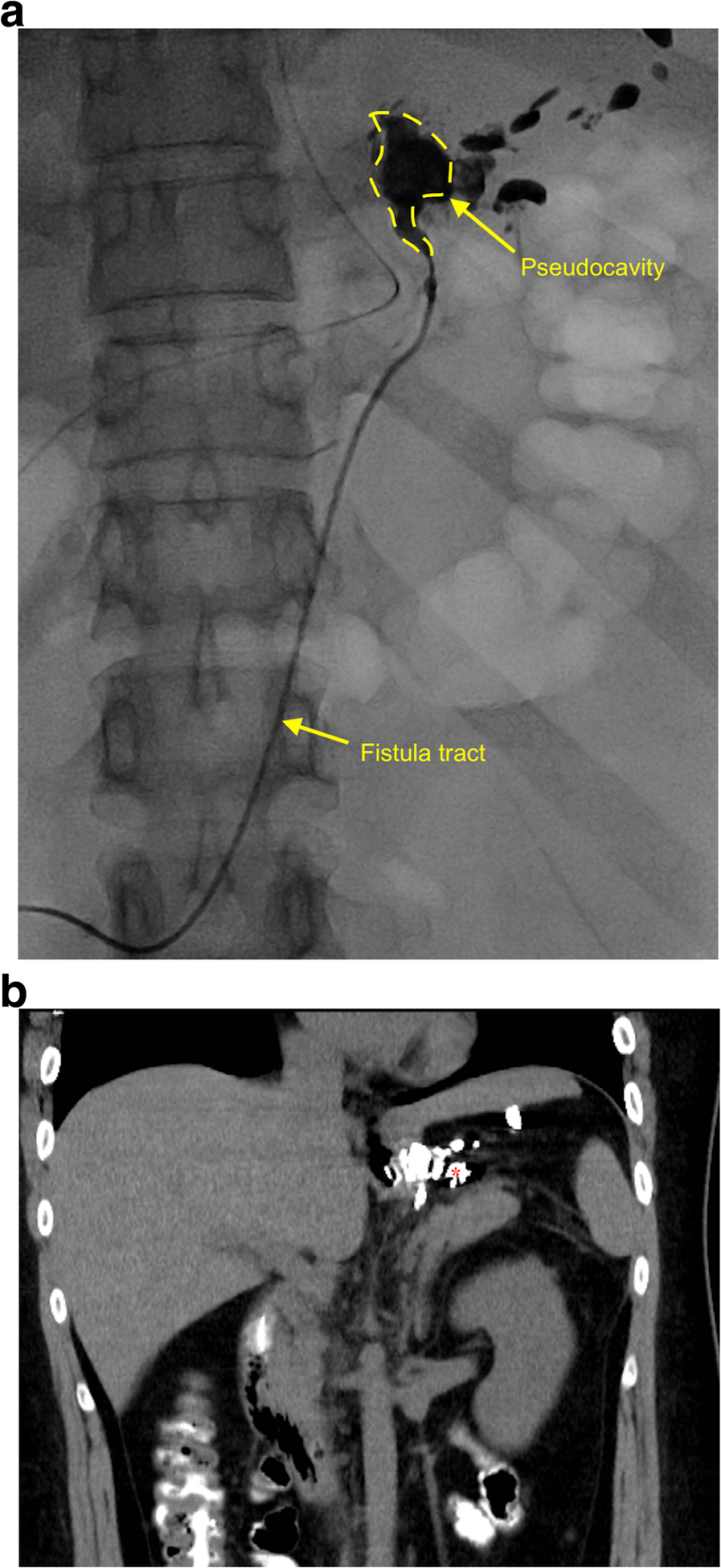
Percutaneous fistula tract embolization was performed through the CWV drain insertion site at POD63 (a) and an oral contrast enhanced CT 5 days later, at POD68, showed the space of pseudocavity was filled with glue and no obvious oral contrast leak was noted (b). Abbreviation: CWV, closed wound vacuum; POD, postoperative day; CT, computed tomography
